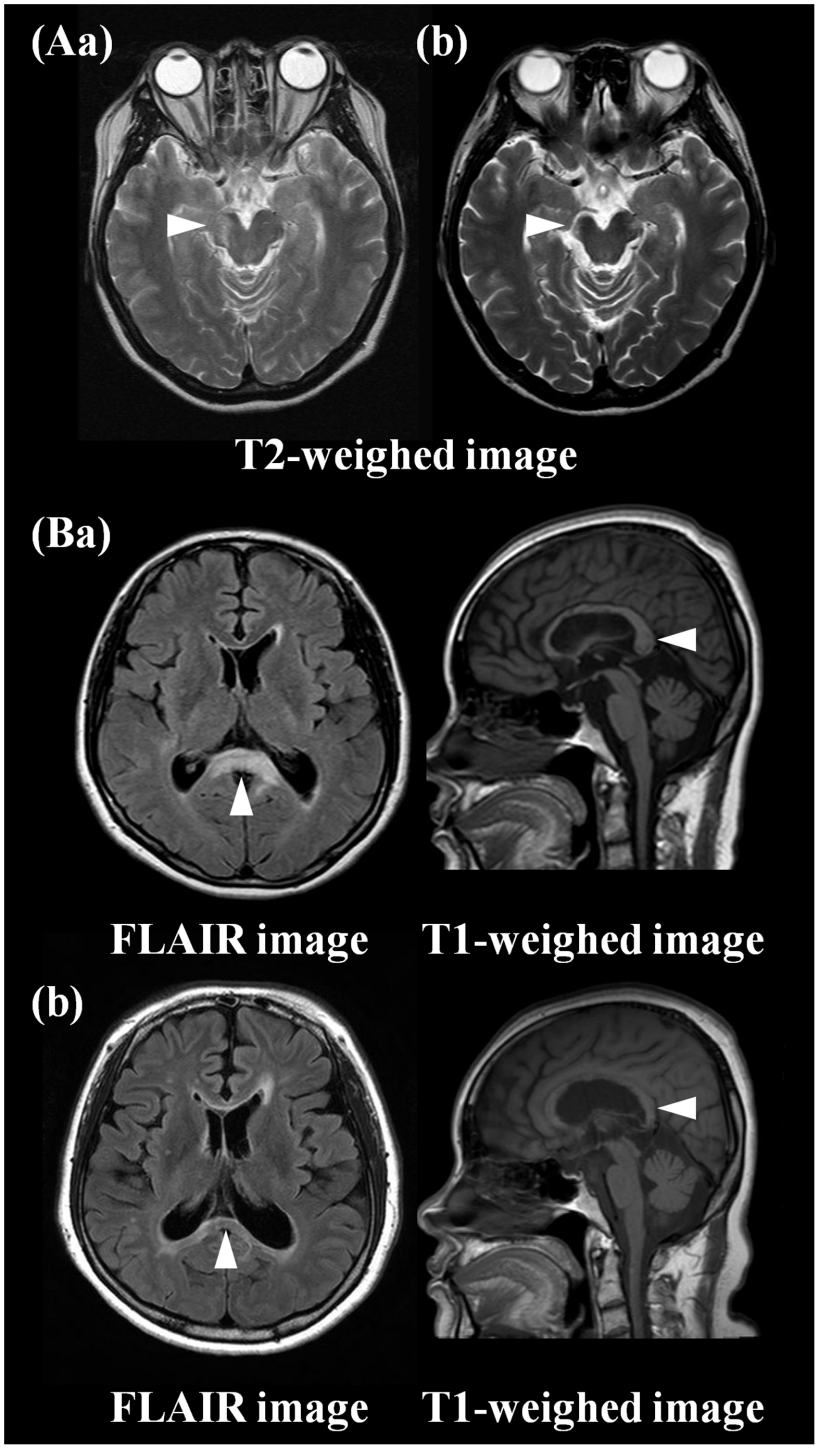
Focal atrophy on follow-up brain MRI. Acute lesions in crus cerebri (Aa) and corpus callosum (Ba) revealed focal atrophy with remnant T2-hyperintense lesions on chronic MRI (Ab, Bb).
